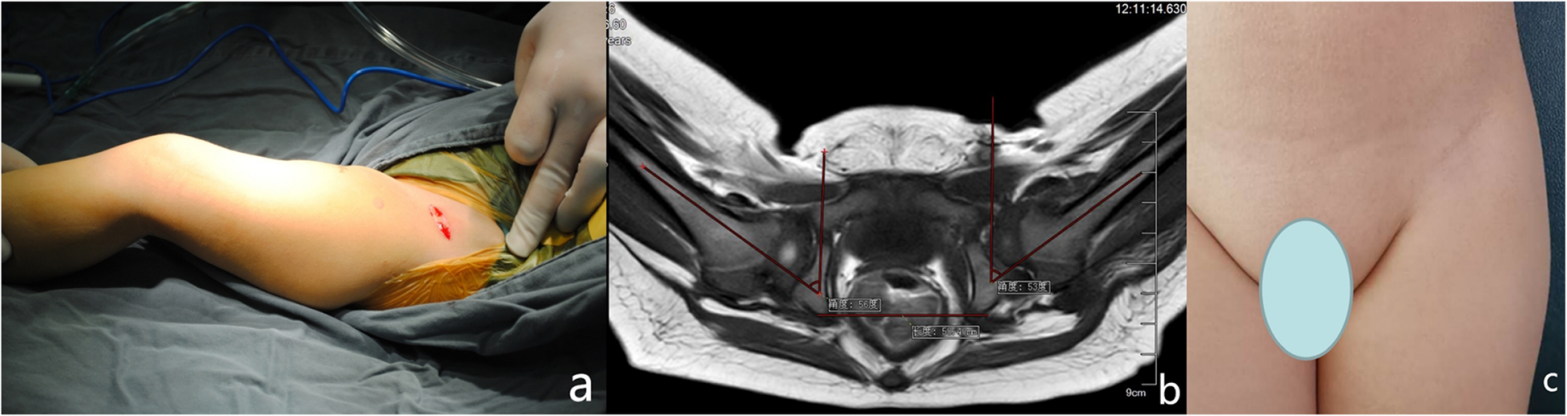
a The incision length was 2.5 cm along the groin crease beneath the ASIS. b MRI confirmed the reduction of the left femoral head. The left hip was in 53° of abduction. c The scar of the incision was almost invisible at 5.5 years after surgery
